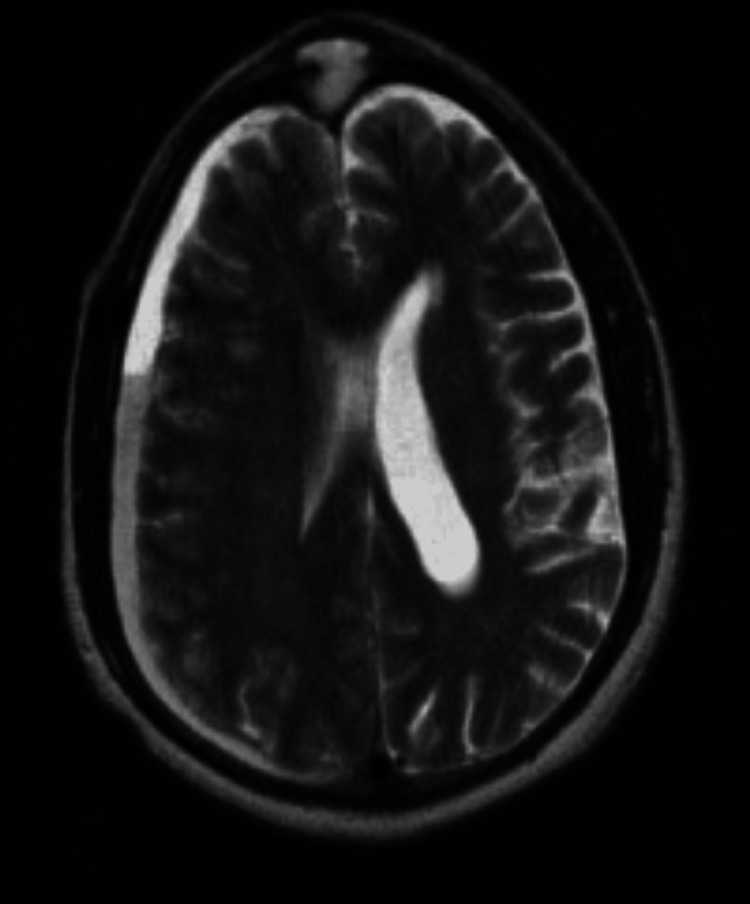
Axial T2-weighted image demonstrating subdural collection with layering effect likely due to settled inflammatory debris or hemorrhage.
